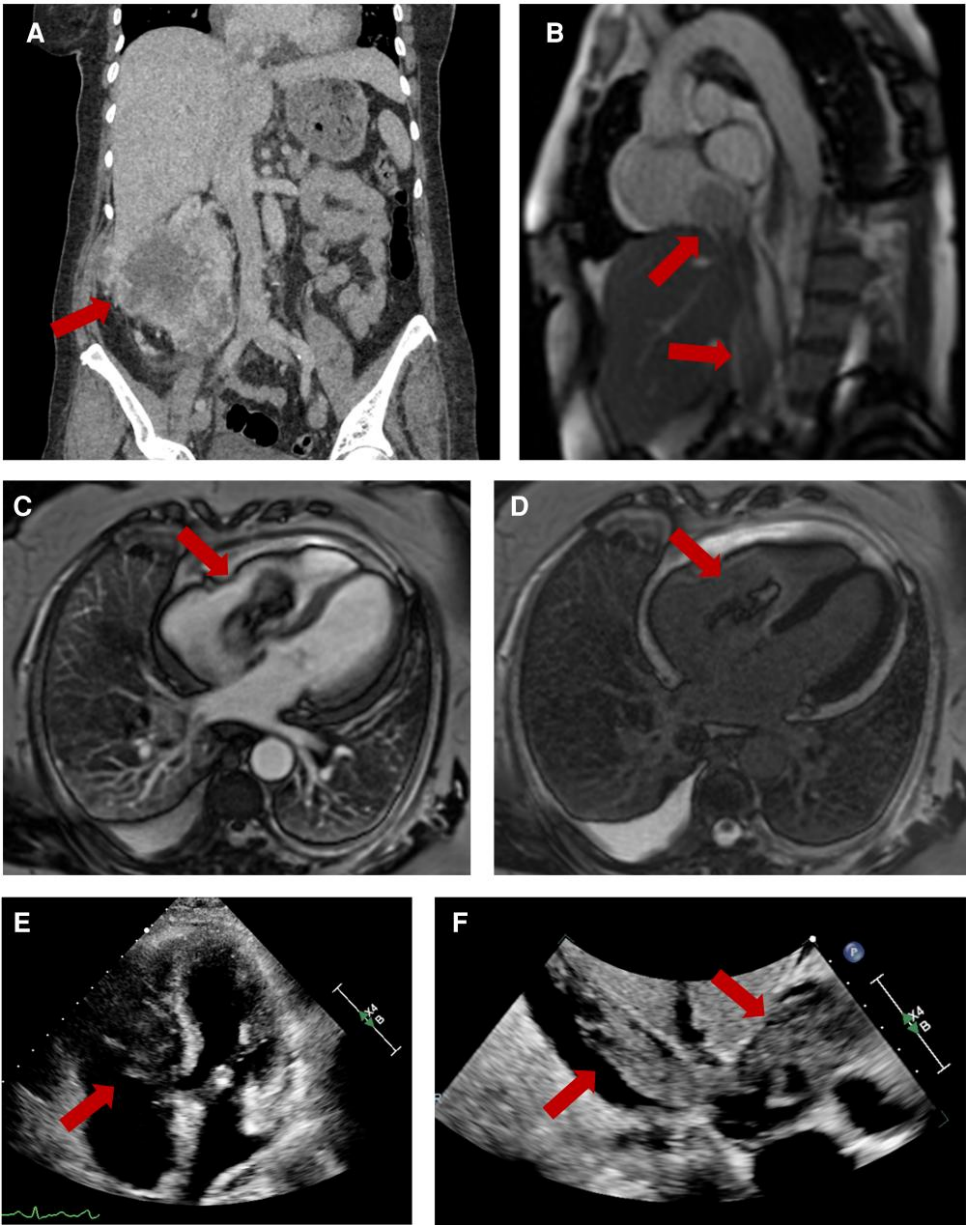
A 71-year-old woman with no significant past medical history presented to the emergency department with a 1-month history of worsening abdominal pain and bilateral lower-limb oedema. Computed tomography of chest, abdomen, and pelvis showed a large right renal mass (12 cm × 10 cm; *Panel A*), multiple lung metastasis, mediastinal, and right hilar adenopathy. Tumour thrombus was seen within the inferior vena cava (IVC) with extension to the right atrium and ventricle (*Panels E and F*). Cardiac magnetic resonance imaging (MRI) demonstrated a large, mobile mass (53 mm × 25 mm) extending along the whole IVC into the right atrium across the tricuspid valve into the right ventricle (*Panels B* and *C*; Supplementary material online, *Video S1*). There was no contrast uptake on first pass perfusion with heterogeneous late gadolinium enhancement in the periphery and central avascular core (*Panel D*). The patient was asymptomatic at diagnosis, with mild–moderate tricuspid regurgitation and preserved biventricular systolic function. She was started on anticoagulation with heparin.
